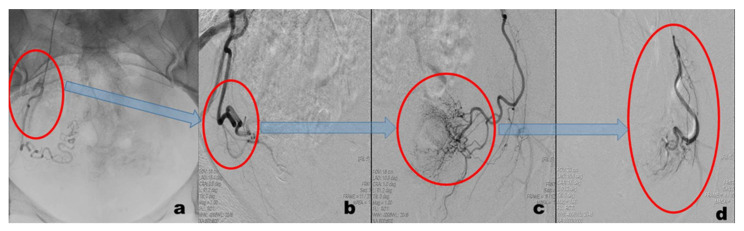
Embolization of the uterine arteries following a few simple steps, defined in the red circle: (a) introduction of a 5F Roberts catheter in the right femoral artery; (b) X-ray guidance of the catheter to the point of embolization; (c) release of polyvinyl alcohol or gelfoam particles to block the blood circulation in the placenta; and (d) blockage of the circulation system in the developing placenta.
